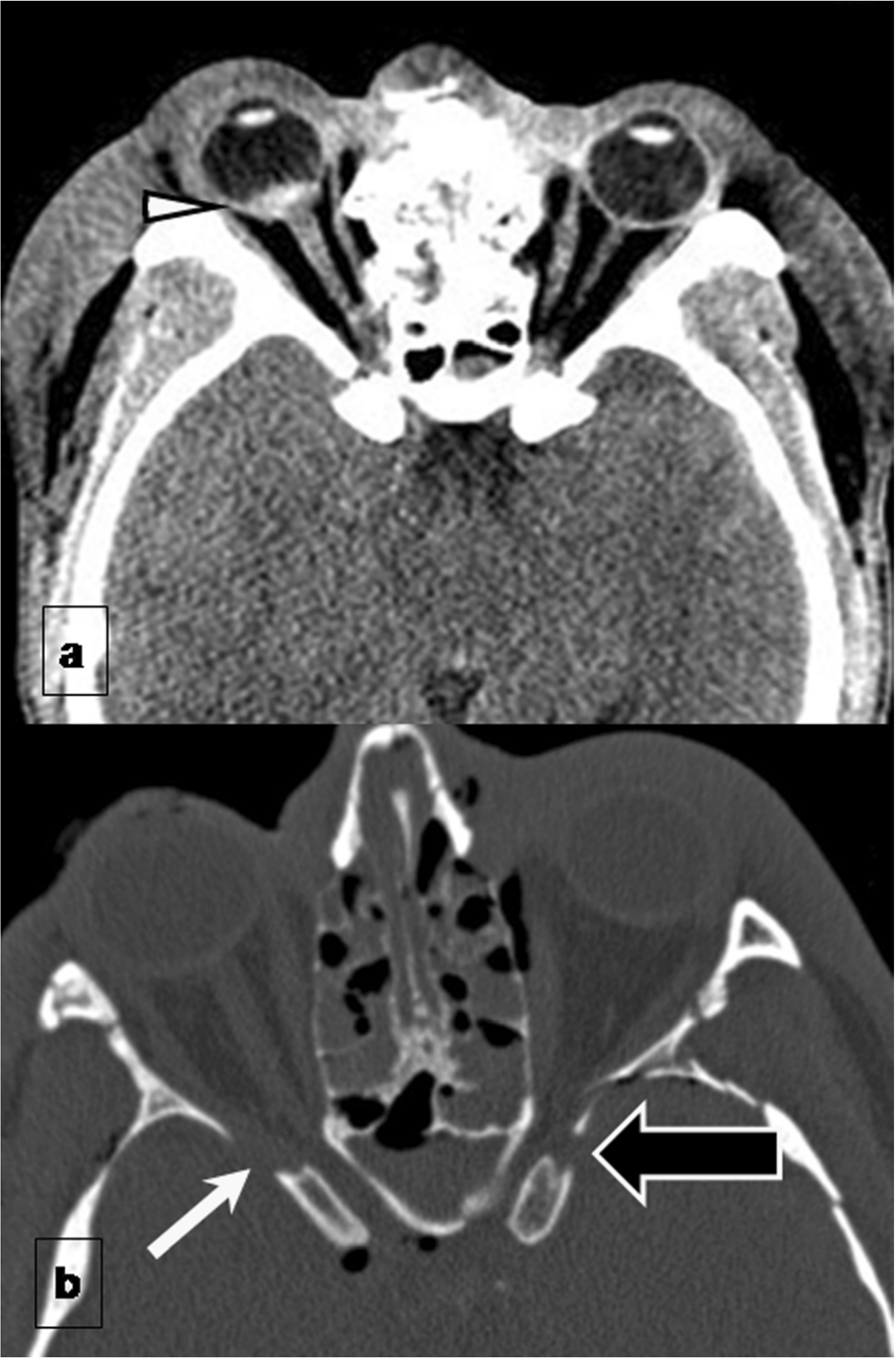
Complications of facial fractures. a Intraorbital complications. Axial unenhanced CT at the level of the mid-orbit shows a hematoma of the posterior pole of the globe affecting optic nerve papilla (arrowhead) in a naso-orbito-ethmoid fracture (not shown). The patient developed traumatic optic neuropathy with right blindness. b Neural foramina complications: superior orbital syndrome. CT shows left superior orbital fissure narrowing (black arrow) with respect to the normal right side (white arrow) by Le Fort III fracture with external wall displacement that can affect CN III, CN IV, CN V1, and CN VI
